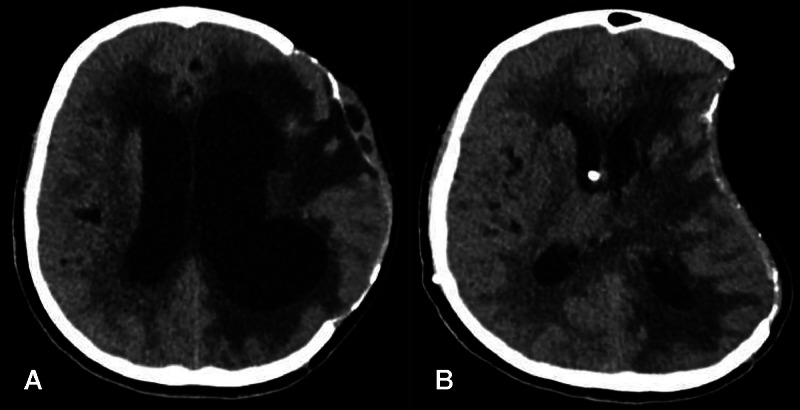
Per and post VP shunt. (A) Non-contrast CT scan of the brain. Due to increased intracranial pressure, an urgent decompressive craniectomy was performed, and a VP shunt was inserted. In (A), the left hemisphere is seen herniating through the craniectomy site. (B) Shows a reduction in pressure after VP shunt insertion." (B) Status post-VP shunt CT scan of the brain. A previous left frontotemporal-parietal craniotomy is noted, along with associated postsurgical changes. A new right transfrontal drain is present, with its tip located within the right lateral ventricle. Small foci of intracranial air are observed, consistent with recent intervention. There has been an improvement in hydrocephalus, with the bifrontal lateral ventricle now measuring up to 51 mm (previously 59 mm).
